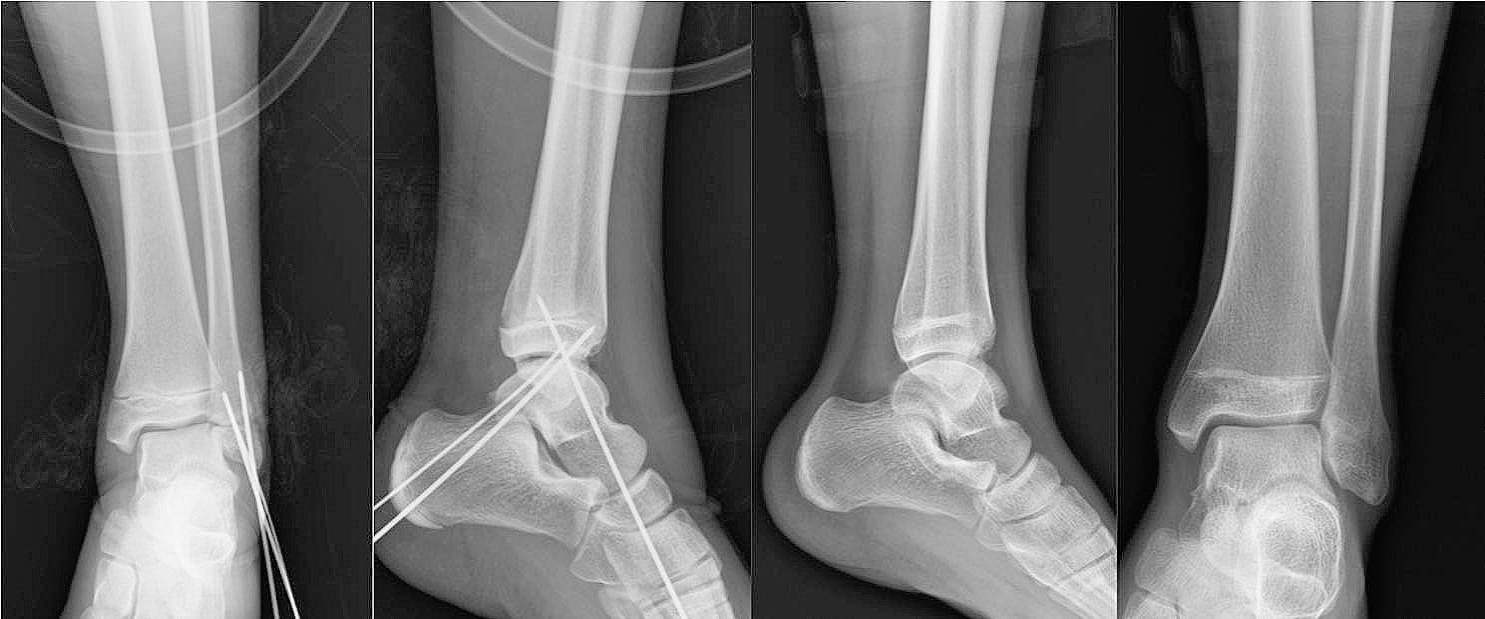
AP and lateral radiographic views of the left ankle during surgery confirming reduction after open reduction and internal fixation and 6 months postoperatively
